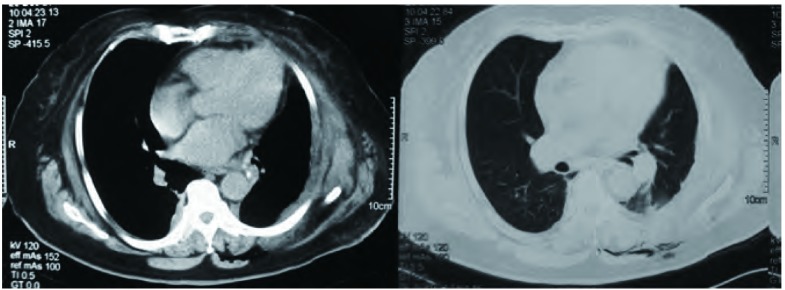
术后复查胸部CT示肿瘤被完全切除 CT scan of the chest showed that the tumor was completely removed after operation
